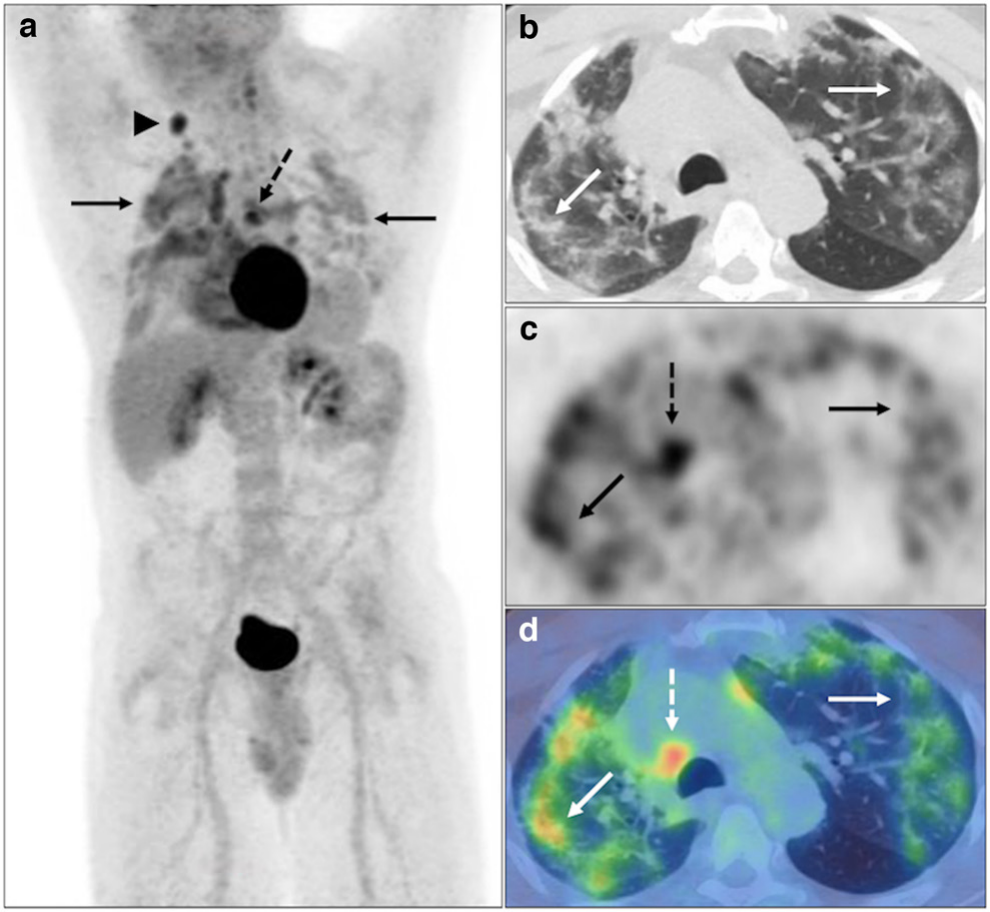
Widespread FDG avid central and subpleural GGO and consolidation with perilobular opacity (solid black and white arrows), *e.g.,* right upper lobe (SUV_max_ 6.7) with reactive borderline normal sized right lower paratracheal (dashed black and white arrows) and right supraclavicular fossa nodes (black arrowhead) on PET MIP (A), axial CT, PET and fused PET-CT (B-D). BSTI 1 without RT-PCR testing (*suspected* case).
